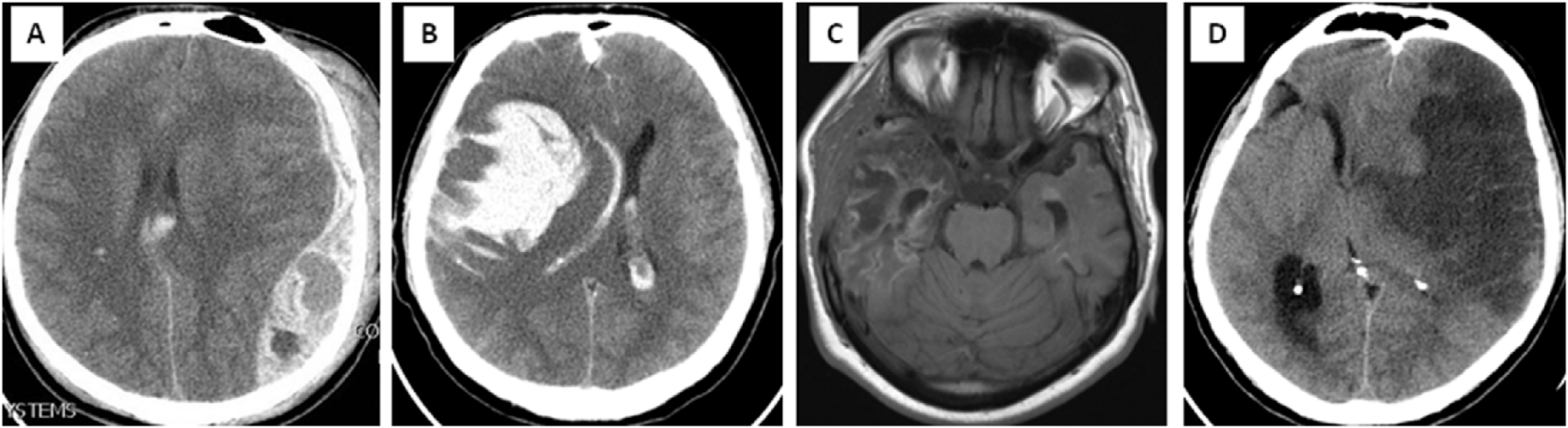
Scans of four representative patients from the study. Patients were treated for a different pathology which caused intracranial hypertension. Three patients were operated on and survived, with residual sequelae, but able to perform daily functions independently. One patient was treated only conservatively, and died. A) Axial CT of a 22-year-old male, with sTBI and traumatic ultraacute ASDH on the left; brain edema and midline shift is visibile; at addmition was GCS 5; urgently treated surgically – hematoma was evacuated and primary DC was performed. B) Extensive intracerebral hematoma in a 37-year-old man with aSAH and edema on the right, caused by a ruptured cerebral aneurysm; at addmission was GCS 4; emergency surgery was done, hematoma evacuated, MCA aneurysm clipped, and Hinge craniotomy was performed. C) Young 26-year-old girl, complication of meningitis and local cerebritis on the left temporal region, followed with thrombosis of middle cerebral artery and stroke; after deterioration of consciousness to GCS 7 she was admitted to neurosurgical department from the infectious disease clinic, and urgently operated - secondary DC was perfomed; early follow up MRI of the brain showed a combination of local infection and insult, with brain fungus temporobasally, at the site of decompression. D) Middle-aged man, 47 years old, transferred from the neurological clinic to the NICU, due to consideration for decompression concerning deterioration of consciousness after malignant MCA stroke verification; GCS was 6; no surgery was done; patient died third day after onset of stroke. (sTBI - severe TBI; aSAH - aneurysmal subarachnoid hemorrhage; ASDH - acute subdural hematoma; MCA - middle cerebral artery)
